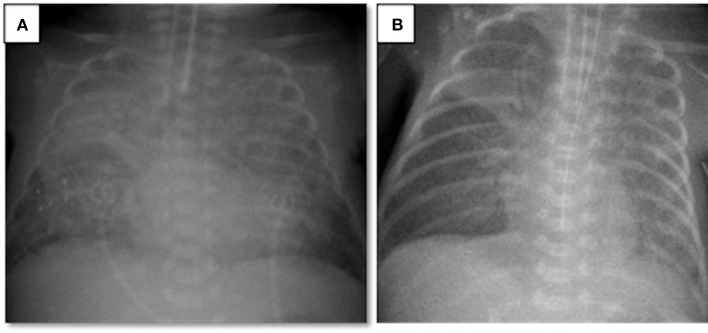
Chest radiograms of Case 1: (A) At the time of ARDS diagnosis, an X-ray revealed bilateral diffuse lung opacities; (B) after two doses of surfactant plus budesonide treatment, a chest X-ray revealed better aeration.
